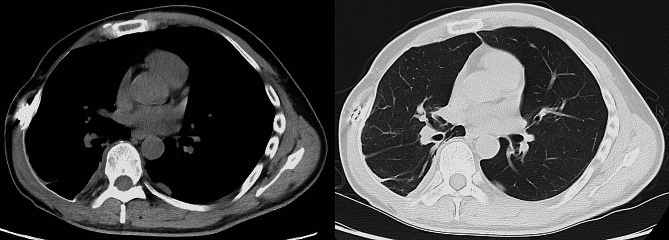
Postoperative CT scan. The combination of titanium plates and Gore-Tex patches creates a three-dimensional thorax
